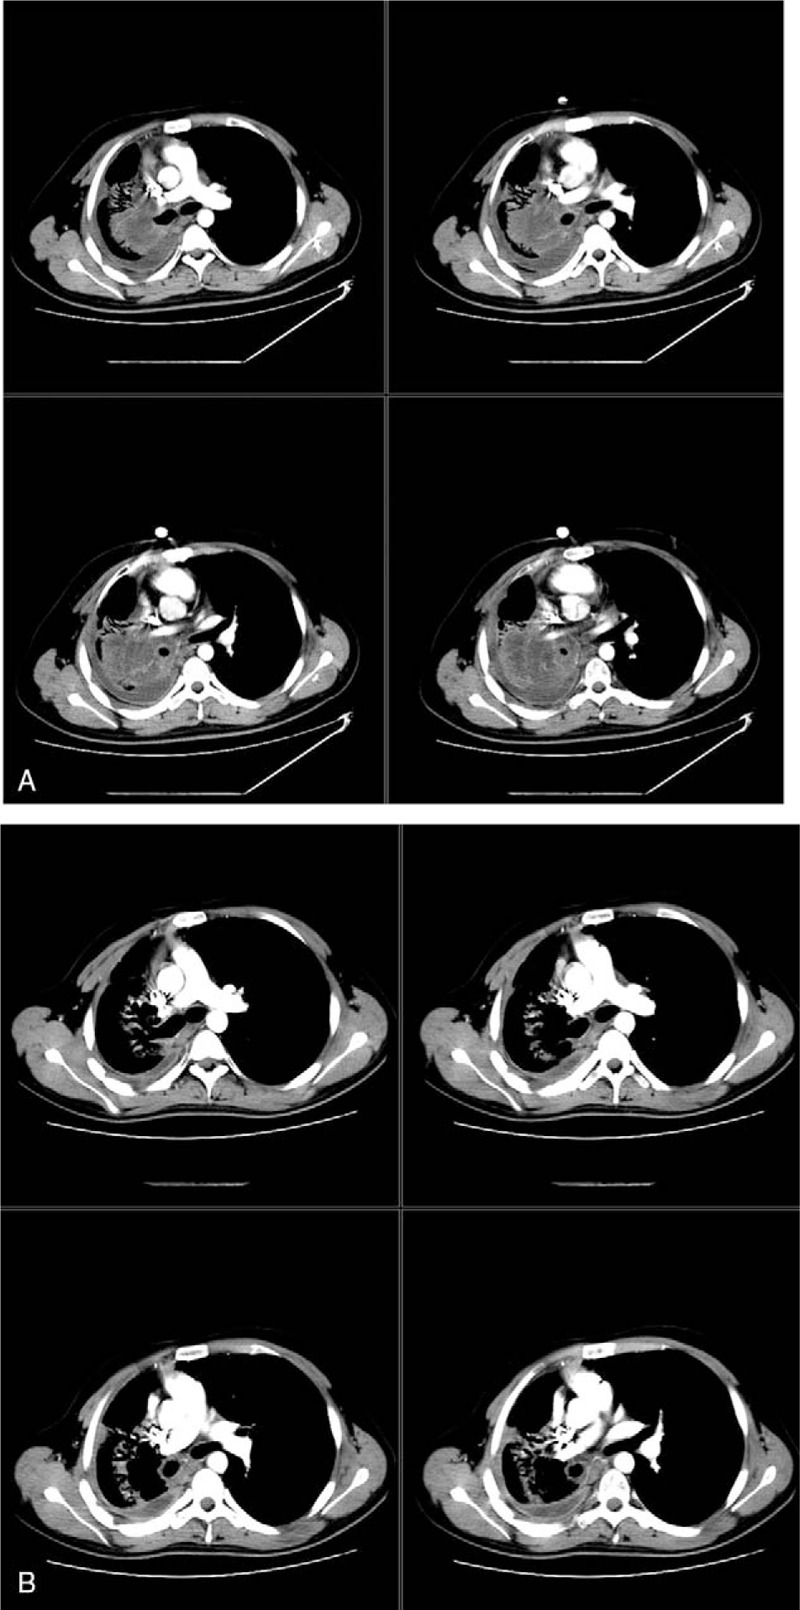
Thoracicoabdominal computed tomography (CT) scan found a soft tissue mass in the right lung. The mass had a maximum diameter of 88 mm × 82 mm. The mediastinum was shifted to the right side (A). The mass was eliminated dramatically (B).
